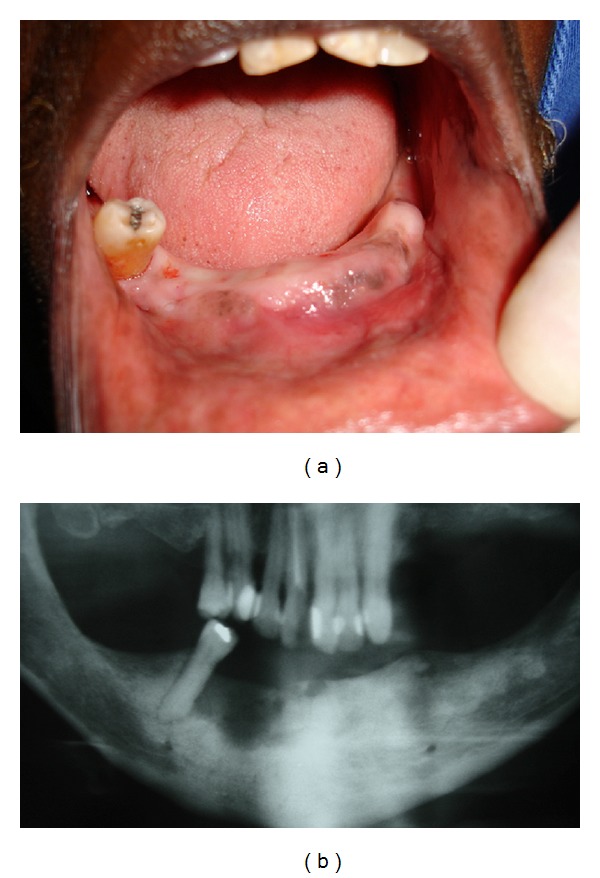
((a) and (b)) Initial clinical and radiographic features of osteomyelitis, located in the anterior mandible, consistent with necrotic bone.
